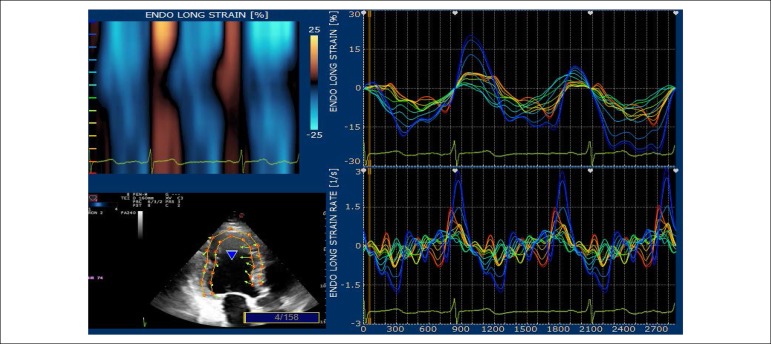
Apical three-chamber – percentage of longitudinal strain in the basal, middle and apical segments of the inferolateral and anterior septal walls.
